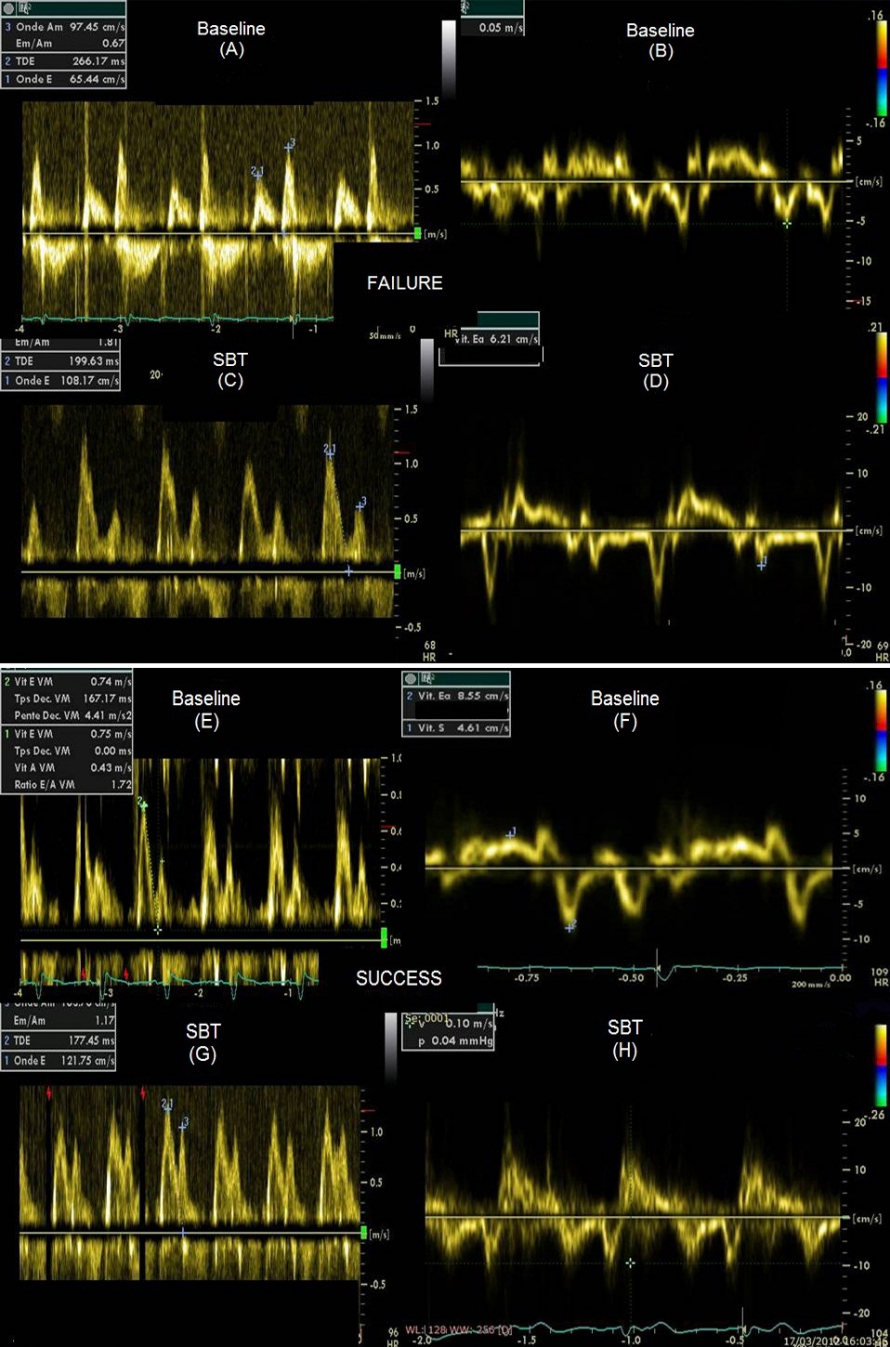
Doppler figure with E/Ea measurement at baseline and during SBT in success and failure. (A) Represents tissue Doppler of mitral annulus at baseline, amplitude of early diastolic wave Ea is 5 cm/sec, the resultant E/Ea ratio is 13. (B) E increases during SBT to 108 cm/sec. (C) Ea is 6.2 cm/sec, the resultant E/Ea increases during SBT to 17.4. (D) Represents pulsed Doppler of mitral flow at baseline in a patient who succeeded, E is 74 cm/sec. (E) Ea is 8.5 cm/sec, the resultant E/Ea is 8.7. (F) E increases during SBT to 121 cm/sec. (G) In the same way Ea increases to 10 cm/sec, then E/Ea ratio remains low during SBT at 12.1.
